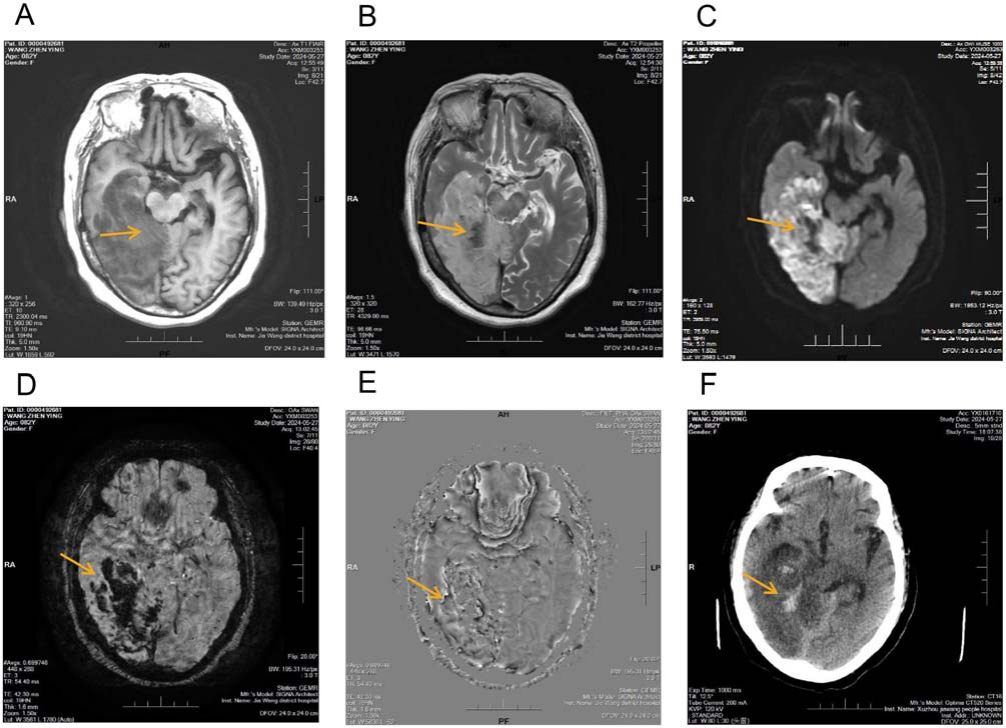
Multimodal imaging findings of acute cerebral infarction with hemorrhagic transformation involving the right frontal, temporal, parietal, and occipital lobes. (A) T1-weighted image demonstrating a large hypointense area corresponding to the infarcted region, without definite identification of hemorrhagic components; (B) T2-weighted image demonstrating extensive hyperintensity consistent with infarction, with internal focal hypointense areas of nonspecific appearance; (C) Diffusion-weighted image demonstrating extensive hyper intensity in the corresponding region, consistent with acute ischemic infarction (yellow arrows); (D) SWI magnitude image showing multiple irregular patchy hypo intense foci within the infarcted area (yellow arrows); (E) Corresponding SWI phase image demonstrating phase alterations at the same locations (yellow arrows); (F) Non-contrast CT showing subtle patchy hyperdensities in the affected region (yellow arrows).
